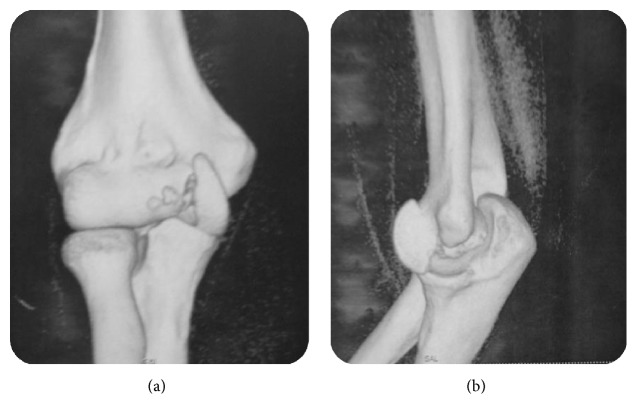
Computed tomography of the elbow with 3D reconstruction showed an isolated shear fracture of the trochlea with no other bony abnormality.
